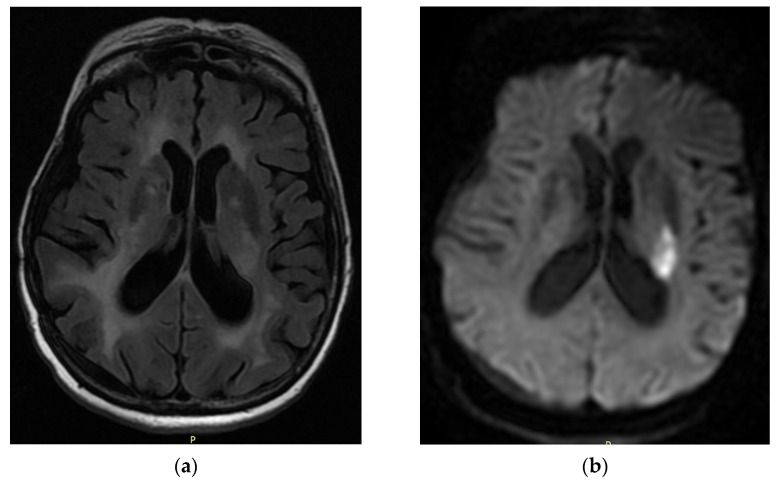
Brain MRI: (a) axial flair and (b) DWI, demonstrating acute ischemic infarction on left basal ganglia, in the distribution area of the left anterior choroidal artery.
